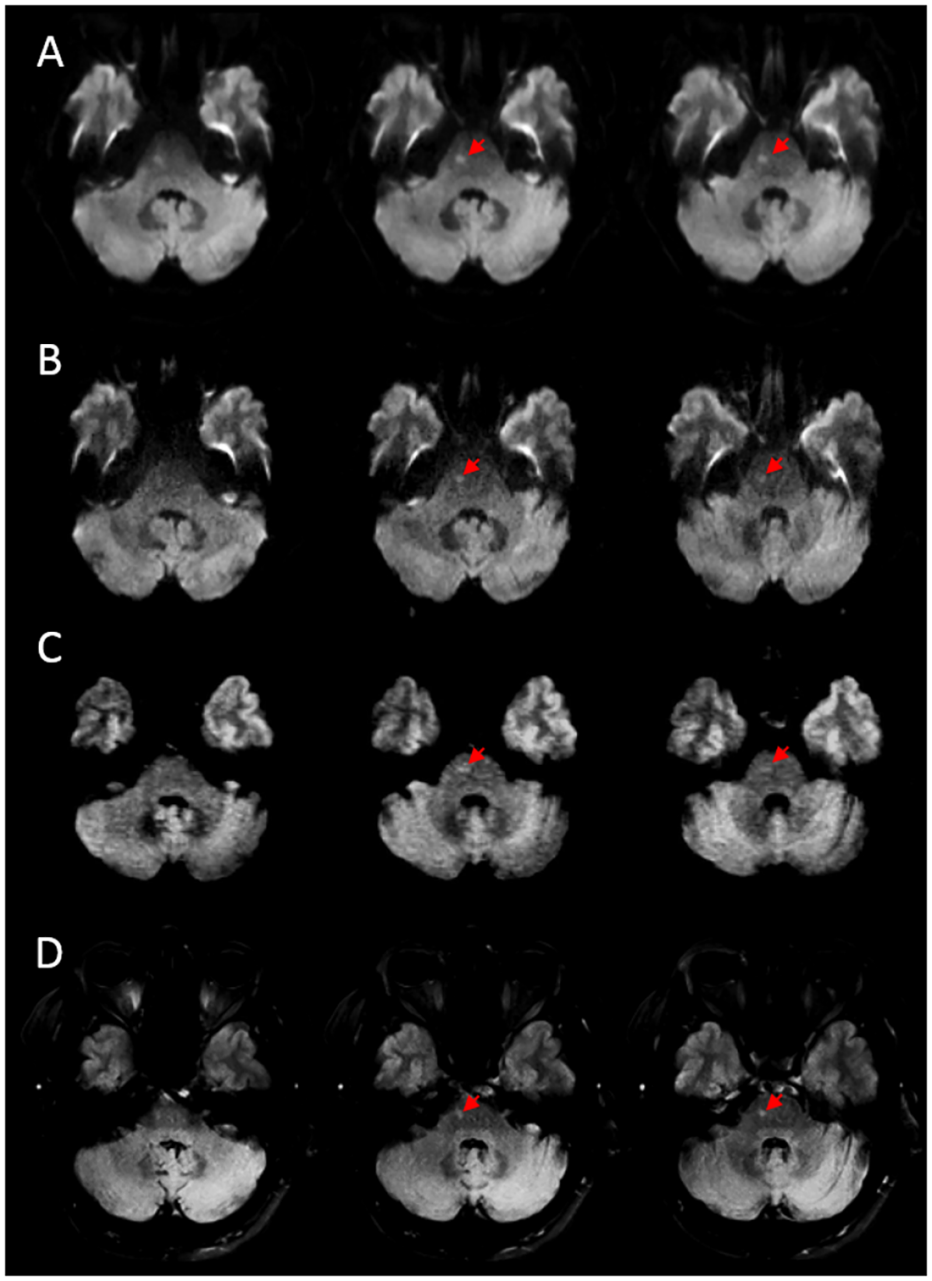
Example of disagreement between DWI sequences for a patient studied 19 hours after onset of stroke symptoms Both low- (A) and high-resolution (B) DWI show a hyperintensity in the right pons that was judged to be an artifact. C) The STEAM-DWI shows a hyperintensity in the right pons that was judged to be an ischemic lesion. D) FLAIR performed 5 days after baseline scans confirmed the presence of an infarct in the same location. Ischemic lesions are indicated by red arrows.
